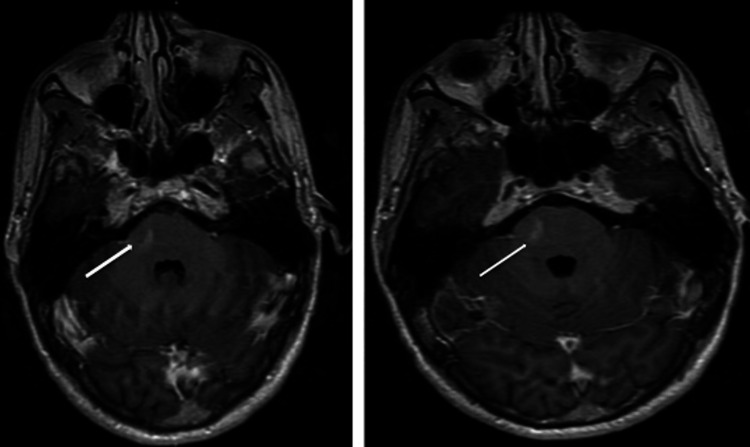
Post-contrast axial T1 MRI images show a focal 1.0 x 0.4 cm area of enhancement in two consecutive sections in the right pons of uncertain etiology.
